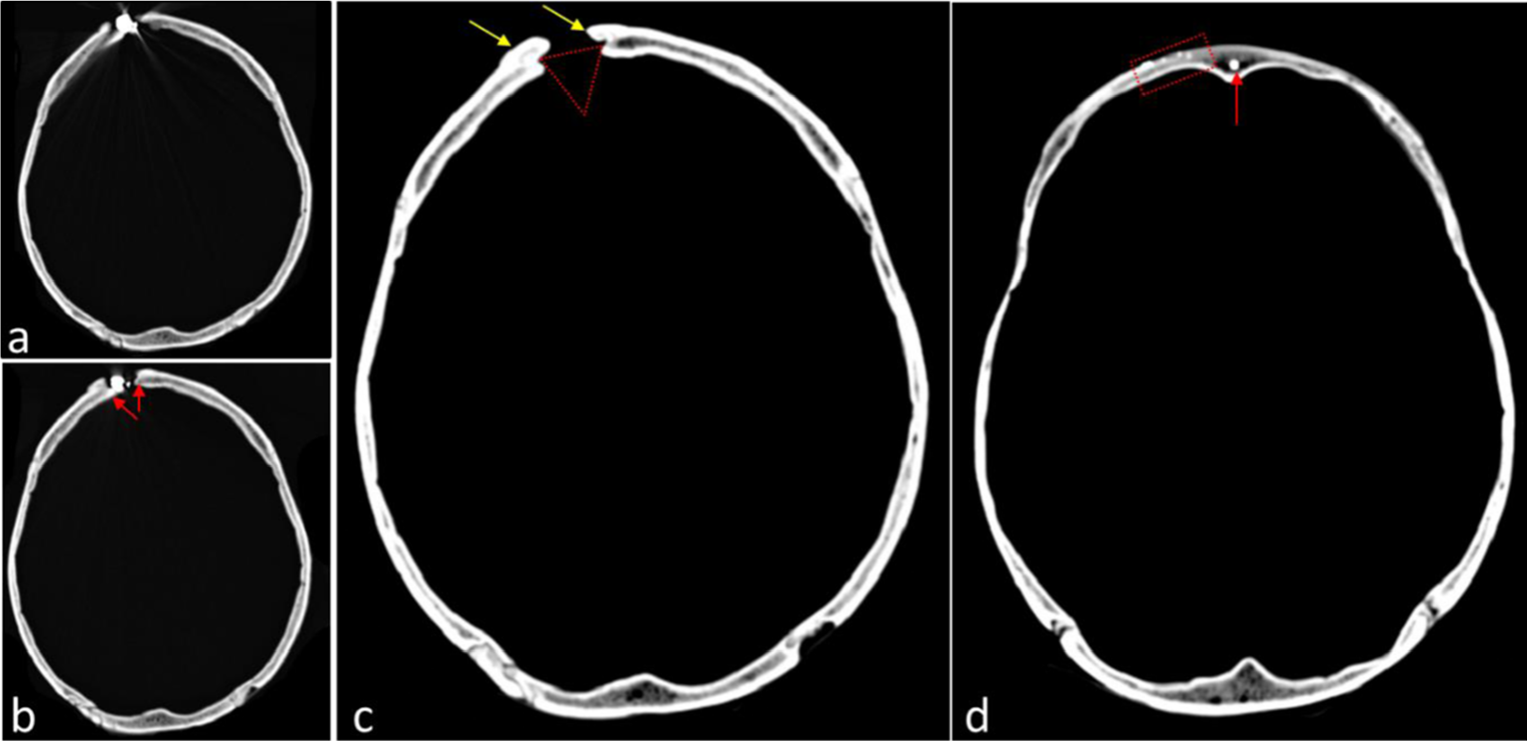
Axial CT images of the cranial vault. 3a and b: image of high radiological density corresponding to the remnants of the firearm projectile (also seen Fig. 2c–e). In Fig. 3b it is also possible to see the image of the Bonet’s funnel (red arrows) and rounded borders, characterizing bone remodelling, evidence of ante mortem healing. 3c: image of Bonet’s funnel in the frontal bone (red triangle). The area of bone remodelling was more intense in the external table of the frontal bone (yellow arrows). 3d: images of high radiological density at the frontal bone (red rectangle) and in the frontal sinus (red arrow) consistent with metallic dust resulting from the firing of a firearm
